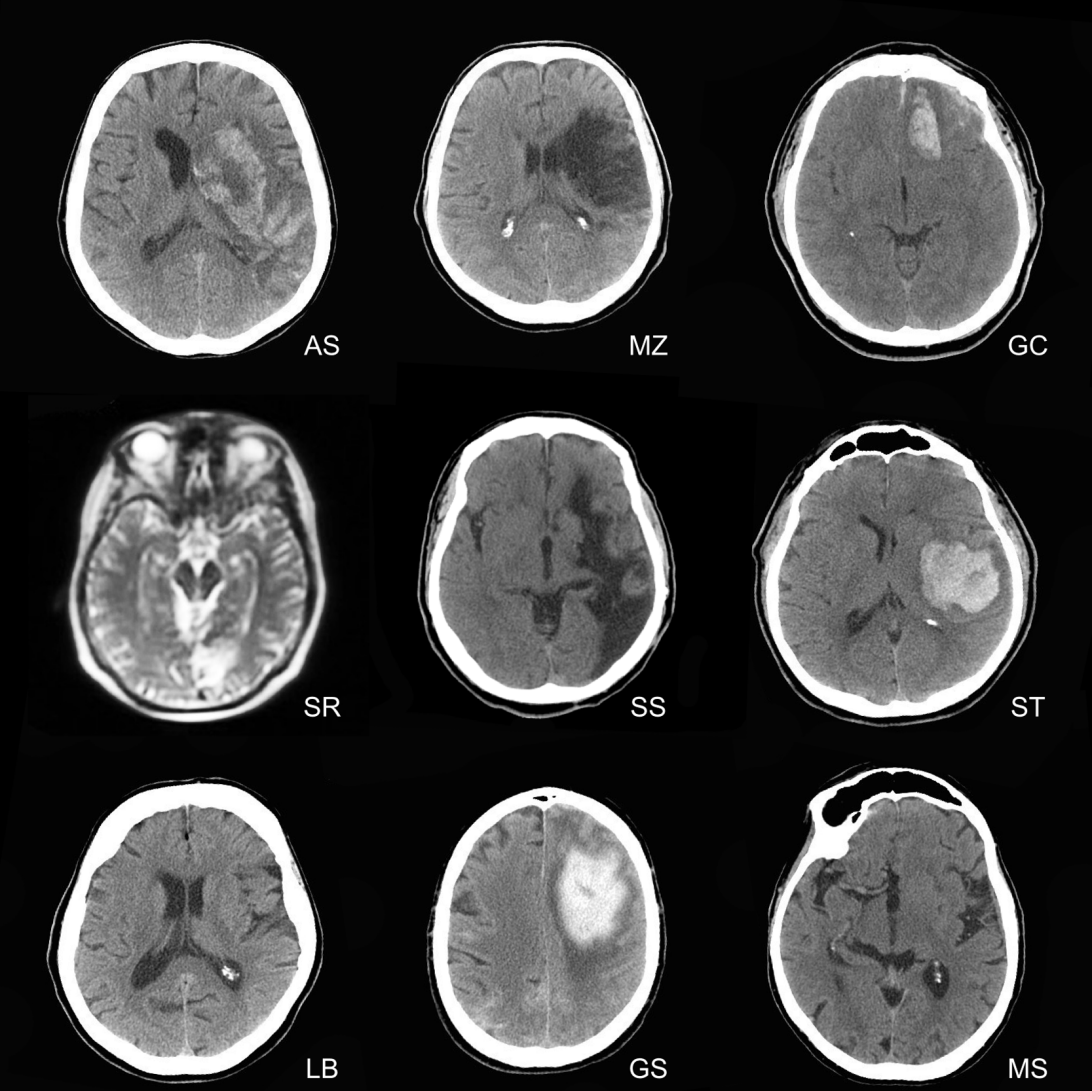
Magnetic resonance imaging and Computed Tomography scans of right hemisphere-damaged patients. The right hemisphere is displayed on the right in each brain scan.
